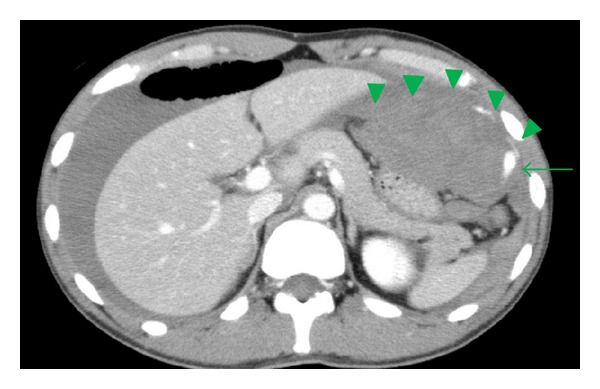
Abdominal enhanced CT revealed a hematoma (arrows) with extravasation (arrowheads) located in the left part of the omentum and relatively high-attenuation fluid in the abdominal cavity.
